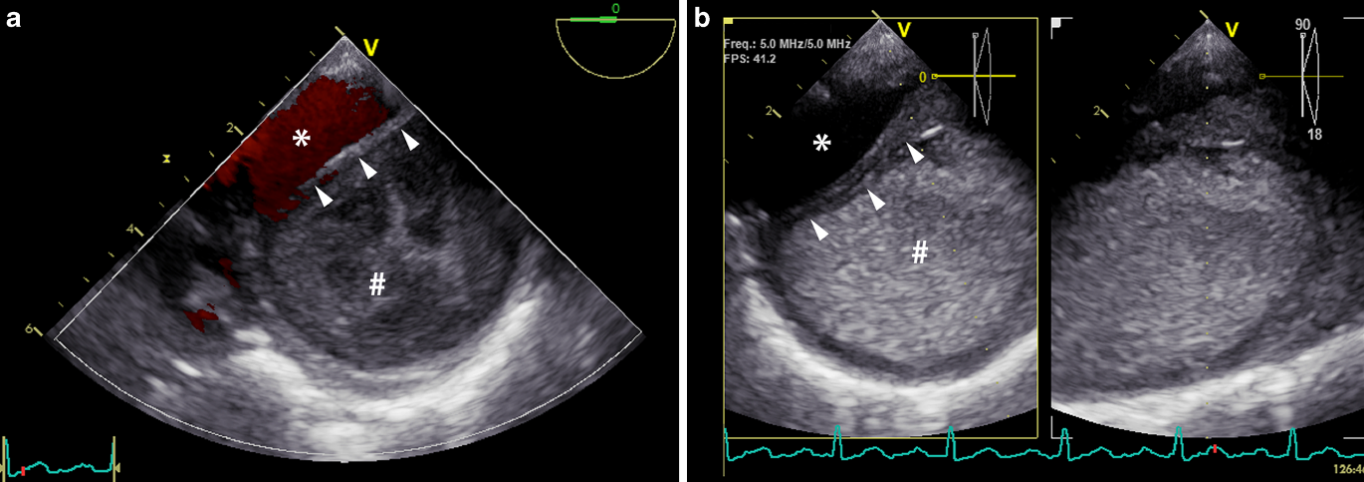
Transverse cross-sectional views of the descending aorta at different levels as documented with TEE. a Transversal cross section of the descending aorta showing two compartments separated by a dissected intimal layer (arrow heads). The largest compartment is the false lumen (hash). Colour Doppler showed laminar flow through the true aortic lumen (asterisk) and no entry site to the false lumen. In the false lumen, slow rotating blood flow existed. See also online video. b Transversal cross section of the aorta more proximally at the aortic arch. Here, slow rotating blood flow in the false lumen was not observed. *Asterisk* true aortic lumen, *hash* false aortic lumen, *arrow heads* dissection layer
